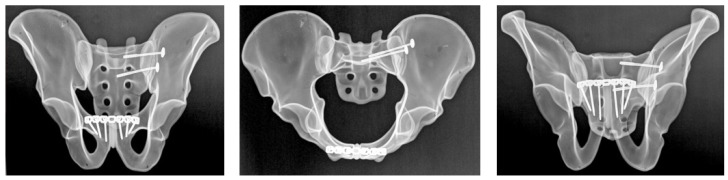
Anteroposterior, inlet, and outlet radiographs showing dual-level sacroiliac screw fixation at the S1 and S2 levels for posterior pelvic ring stabilization.
